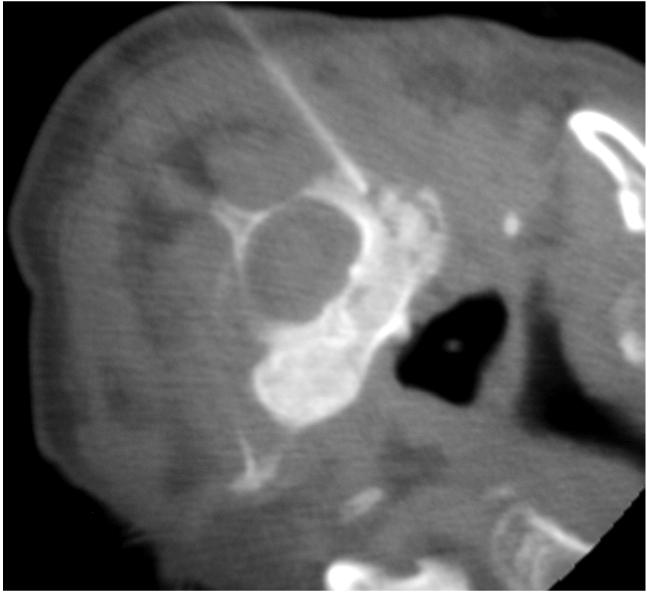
Biopsy of the C2 vertebra in a 61-year-old man with nasopharyngeal carcinoma complicated by C2 vertebral osteomyelitis. Axial CT fluoroscopic image taken with the patient in a lateral decubitus position shows the tip of the Ostycut needle within the right C2 lateral mass. A right posterolateral approach was used.
